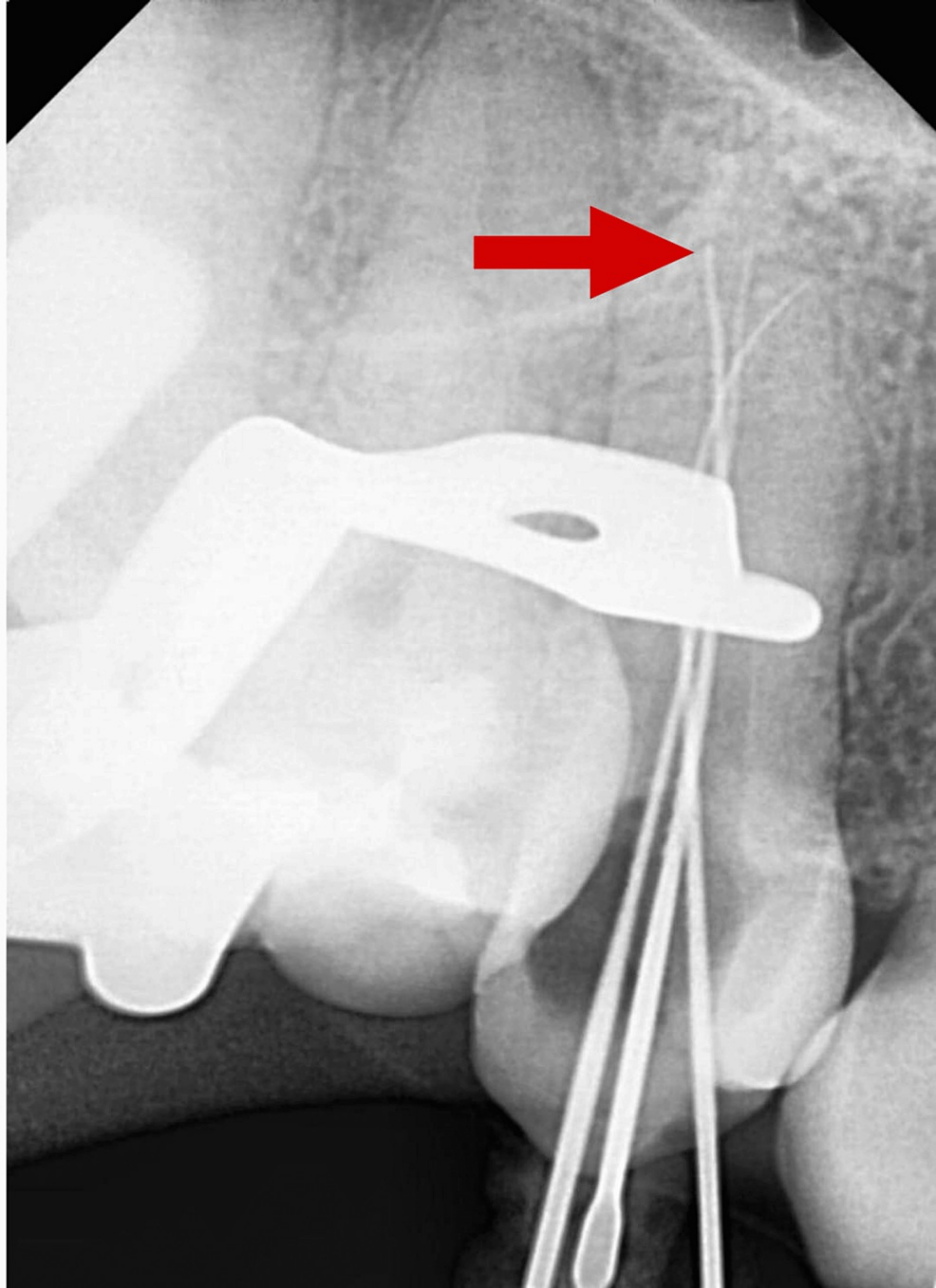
Working length radiograph Arrow depicting distobuccal canal
